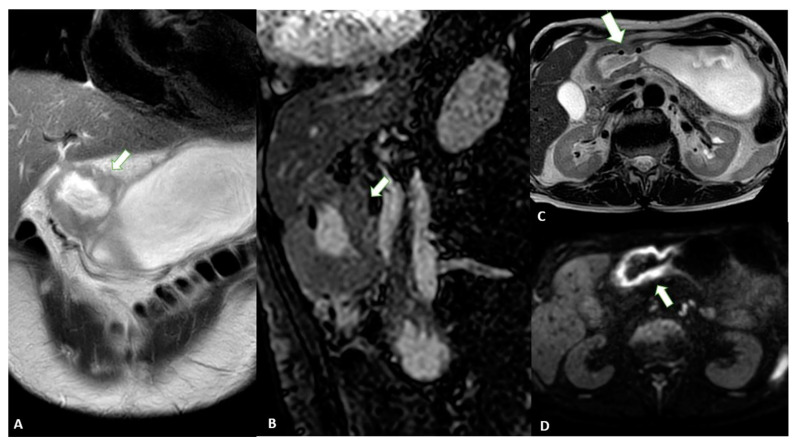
MRI images of a T3 gastric cancer of the gastric antrum in a 79-year-old male patient. (A) Coronal 2D image and (C) Axial 2D image of the Turbo Spin Echo (TSE) T2 sequence showing a circumferential lesion (arrow) invading the subserosa layer with an intermediate signal intensity; (B) Coronal 2D Balance Fast Field Echo (BFFE) sequence showing a circumferential lesion (arrow) with a low signal intensity; (D) Axial Diffusion Weighted Image (DWI) showing an area of signal restriction (arrow) corresponding to the tumor.
